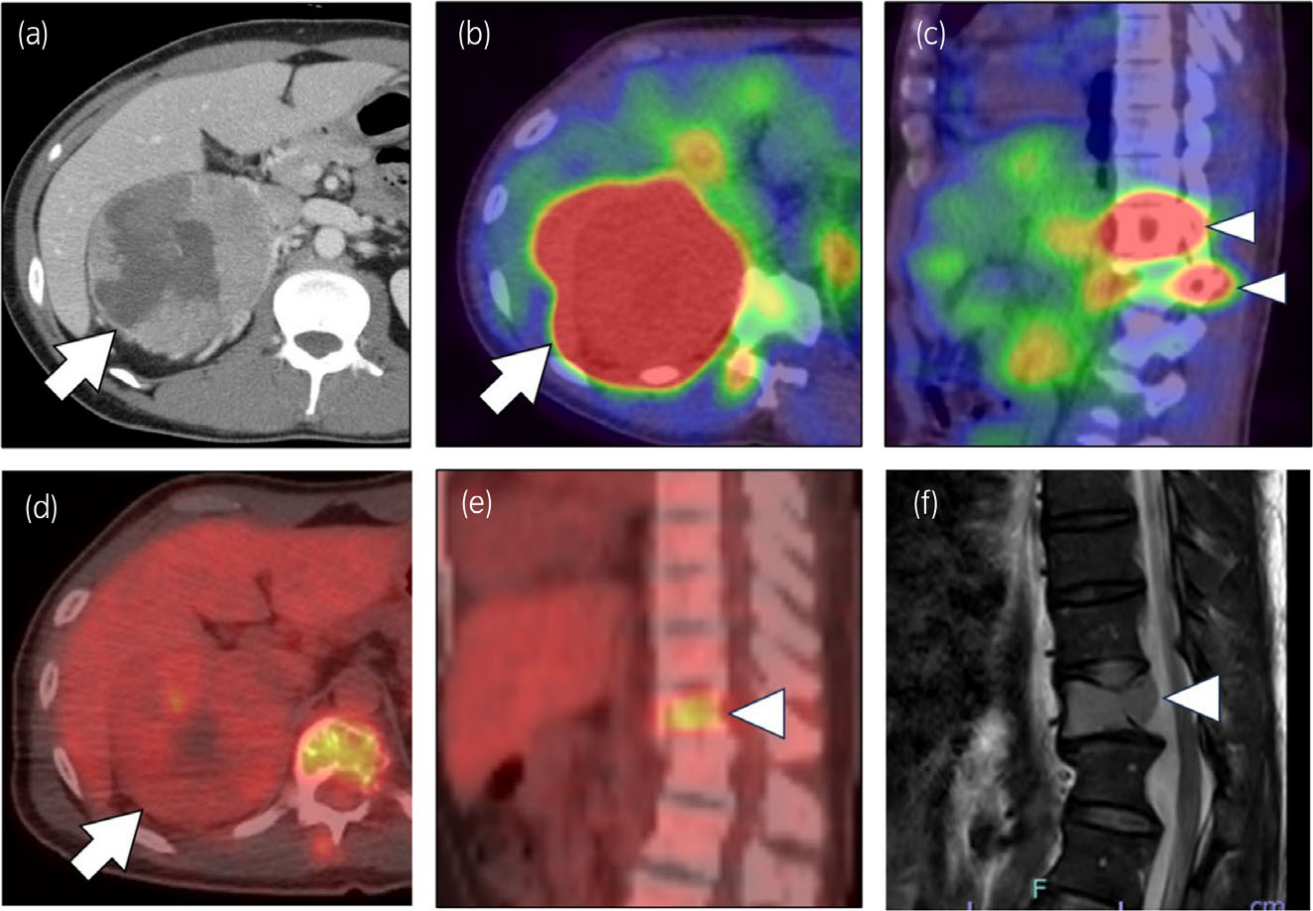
(a) Abdominal contrast CT shows a right adrenal mass (arrow) of 93 mm in size with internal hemorrhage and necrosis. (b, c) 123I‐MIBG scintigraphy shows multiple accumulations in the right adrenal mass (arrow) (b), 11th thoracic vertebral body, and 12th thoracic vertebral arch (arrowheads) (c). (d, e) FDG‐PET CT scan shows accumulation in the adrenal mass (SUVmax 4.0) (arrow) and thoracic vertebra (SUVmax 5.3) (arrowhead). (f) MRI T2‐weighted sagittal image shows loss of normal fatty marrow and high signal in the 11th thoracic vertebra (Arrowhead), with dural sac dilatation at the level of the 11th and 12th thoracic vertebra.
